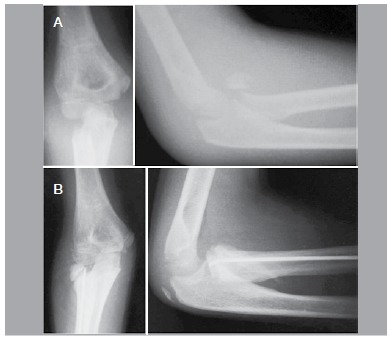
(A) Preoperative x-ray; (B) Postoperative x-ray with signs of osteonecrosis of O'Brien type III fracture.
